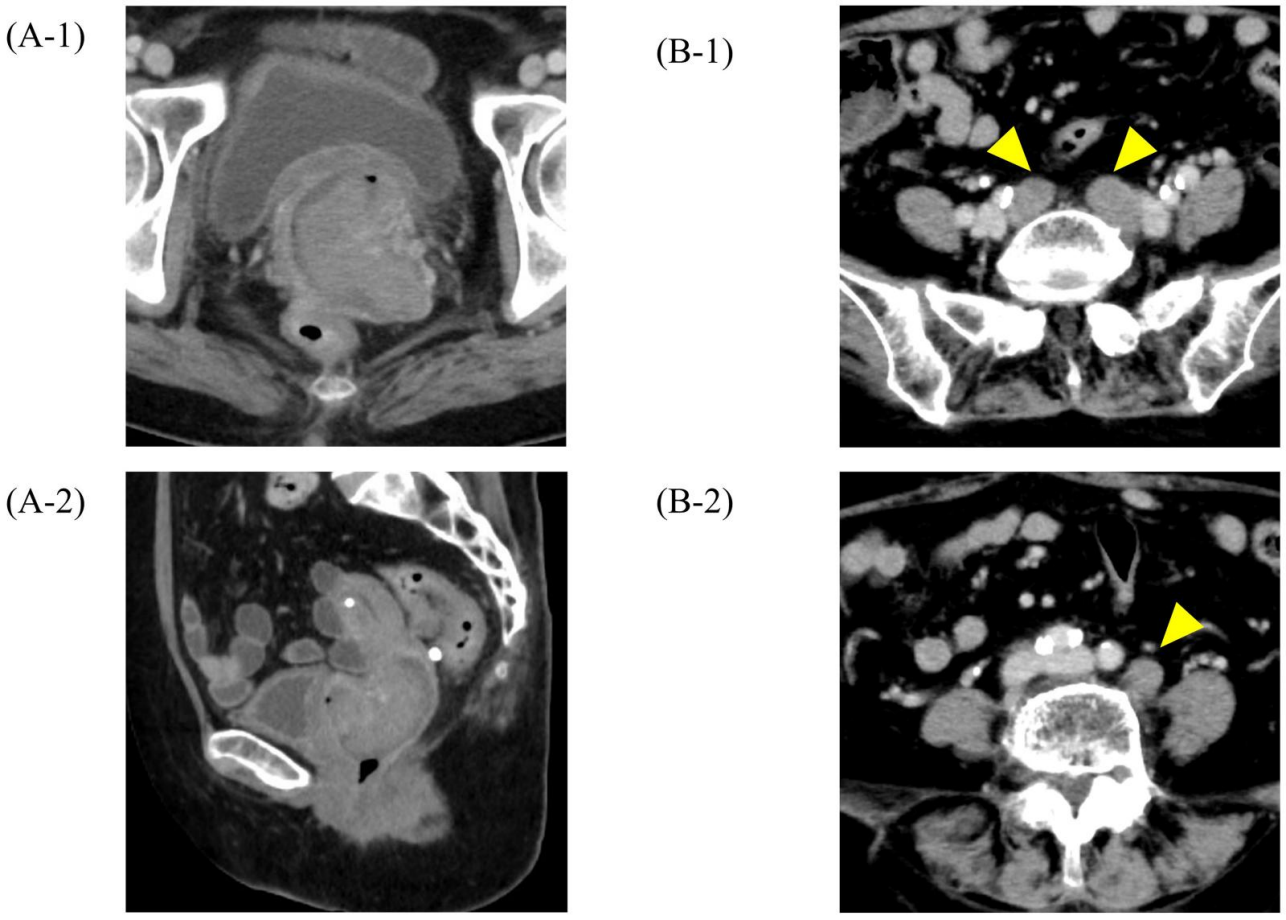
Contrast-enhanced CT images after 2 cycles of chemotherapy. (A-1) Axial and (A-2) sagittal plane images. The cervical mass had rapidly increased to 75 mm in size. (B-1) Axial CT image at the pelvic level and (B-2) at the para-aortic level taken 7 months after the completion of brachytherapy, showing recurrent lymph node metastases (arrowheads). These recurrent lesions were located outside the previously irradiated field.
